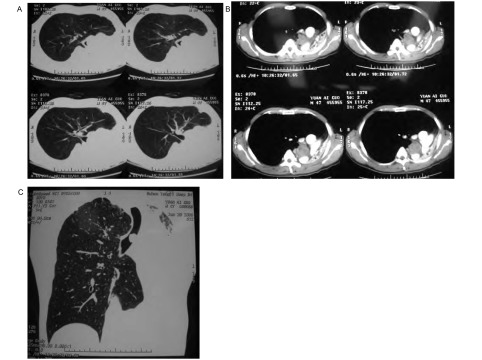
术前CT检查示气管近隆突处见肿块影，左主支气管开口无显示，左全肺不张，右肺过度扩张 CT scan before operation showed mass in trachea near carnia, the openning of left bronchea wasn't showed, left lung collapsed, and right lung over expanded
